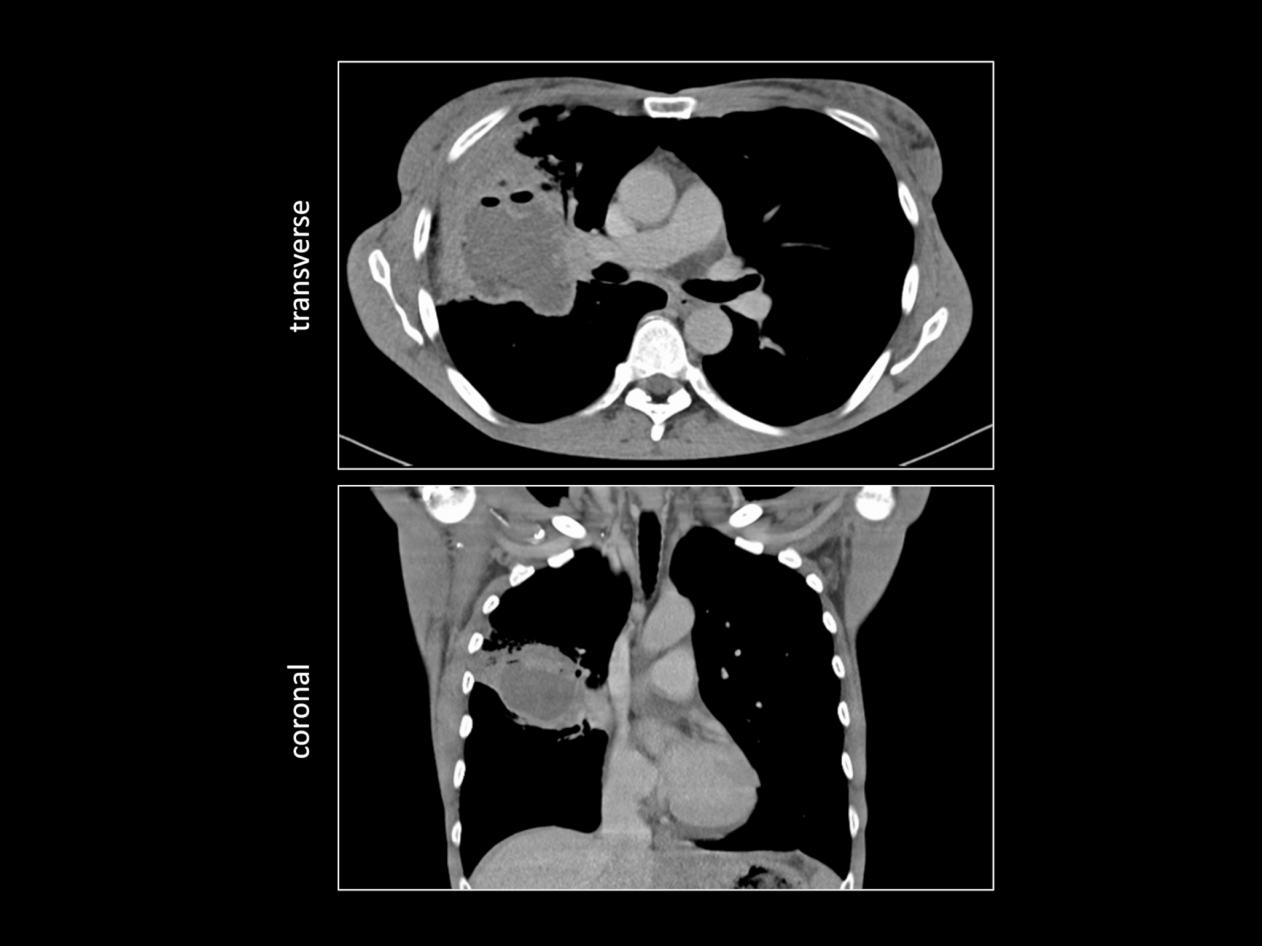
Chest CT scans in the soft tissue window demonstrating the lung abscess. CT imaging was done two weeks after admission, when lung abscess first became apparent. Two representative images in transverse and coronal plane are demonstrated
